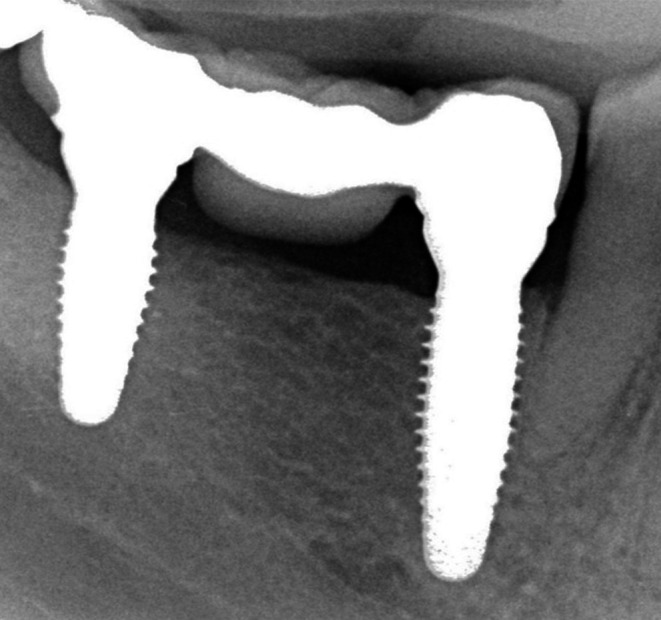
Corresponding radiograph of the peri‐implant bone situation of the two implants supporting an FDP at the 10‐year follow‐up.
